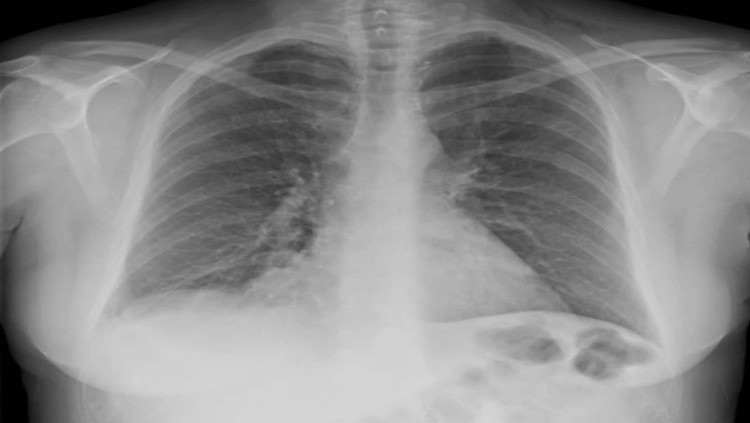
Immediate postoperative chest X-ray was normal
